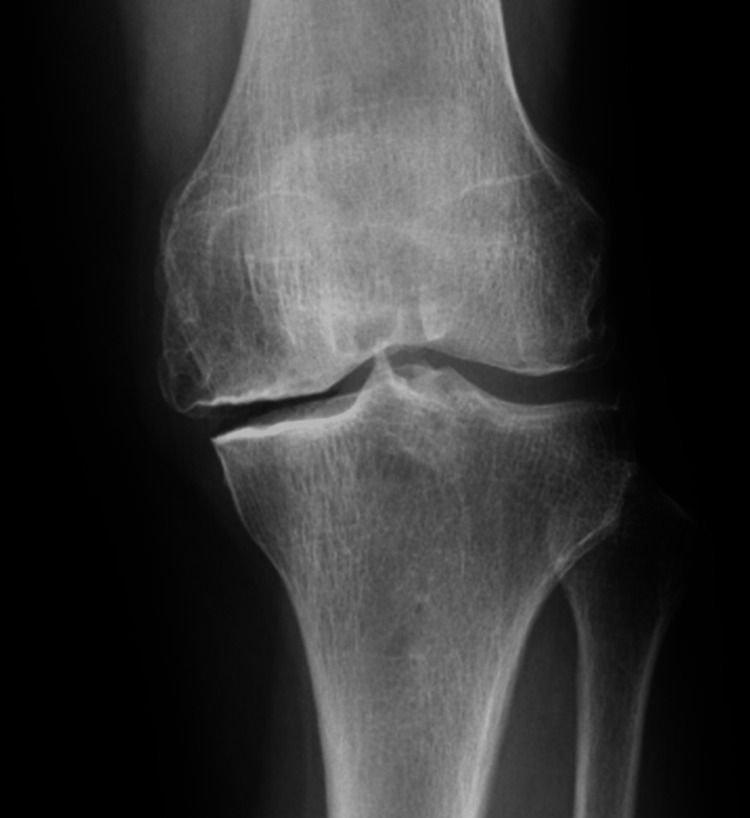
Preoperative radiography
